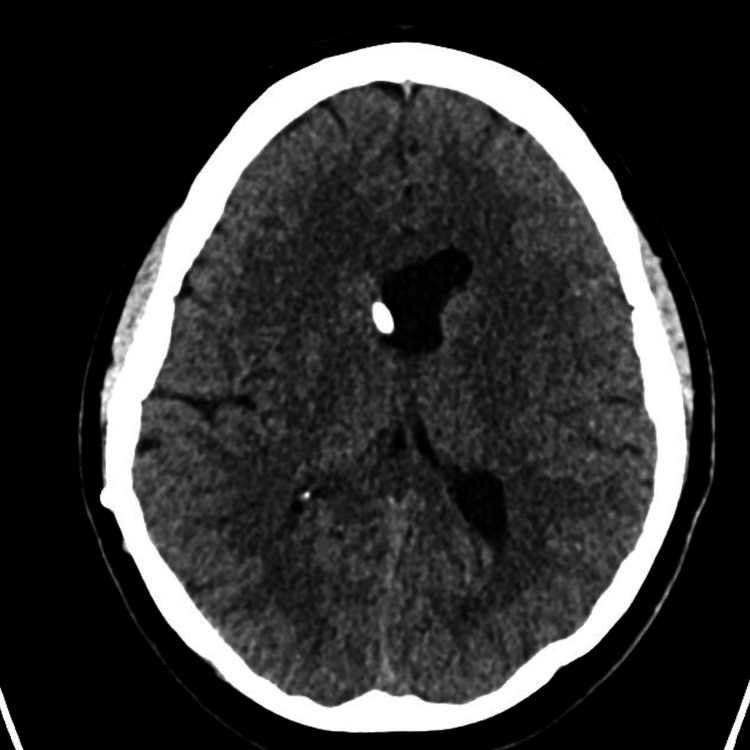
Noncontrast CT brain This figure shows a noncontrast CT of the brain in July 2025, demonstrating the patient's VP shunt in the lateral ventricle. CT: computed tomography, VP: ventriculoperitoneal
